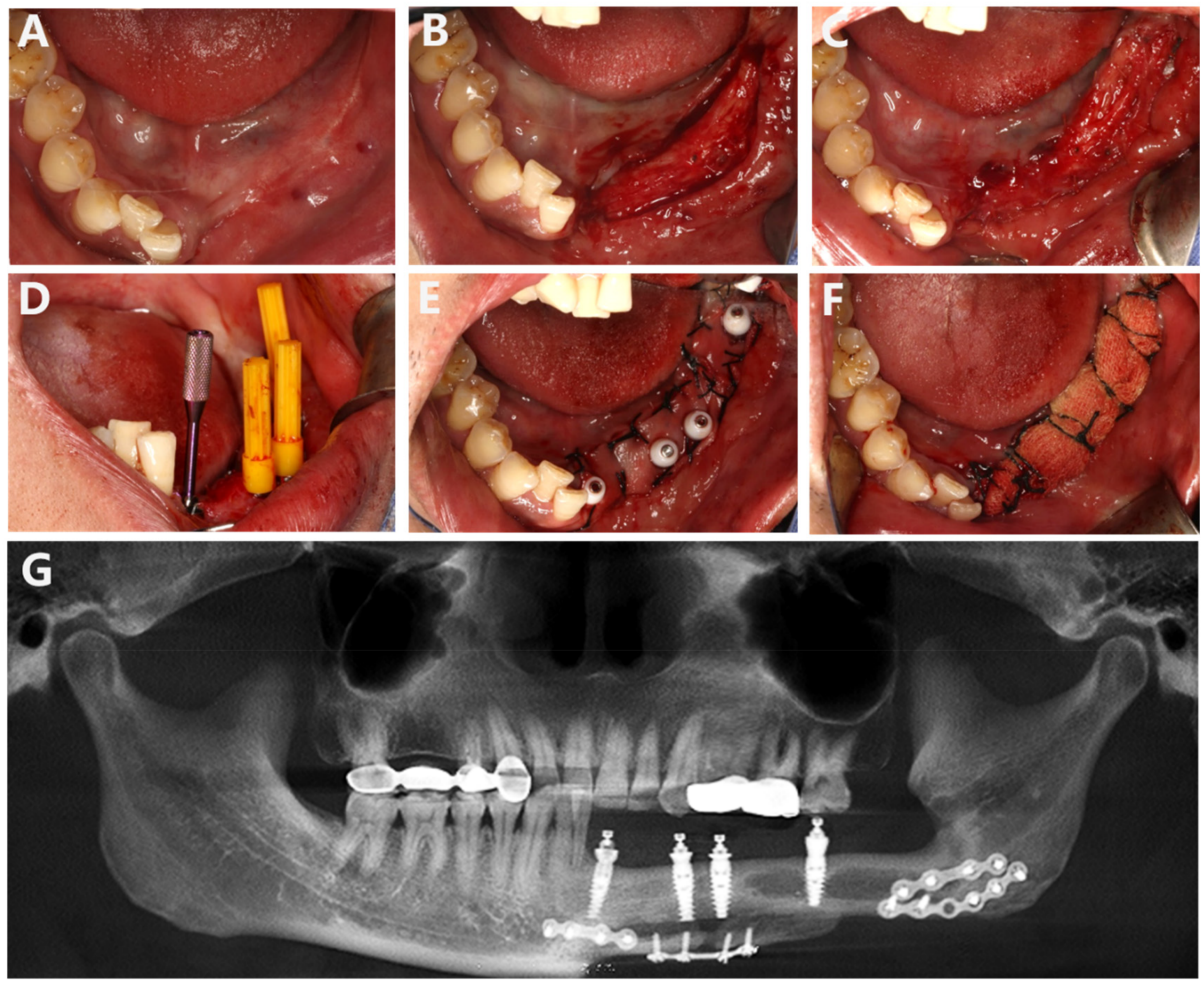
(A) Preoperative mucosa at the second stage of implantation. (B) Incision of the gingiva. (C) Fixation of local mucosa to the buccal and lingual periosteum. (D) Placement of the Multi-unit abutment. (E) ADM implantation. (F) Local pressure stabilization with iodoform gauze strips. (G) CBCT imaging after placing the Multi-unit abutment indicates that the abutment is properly positioned.
